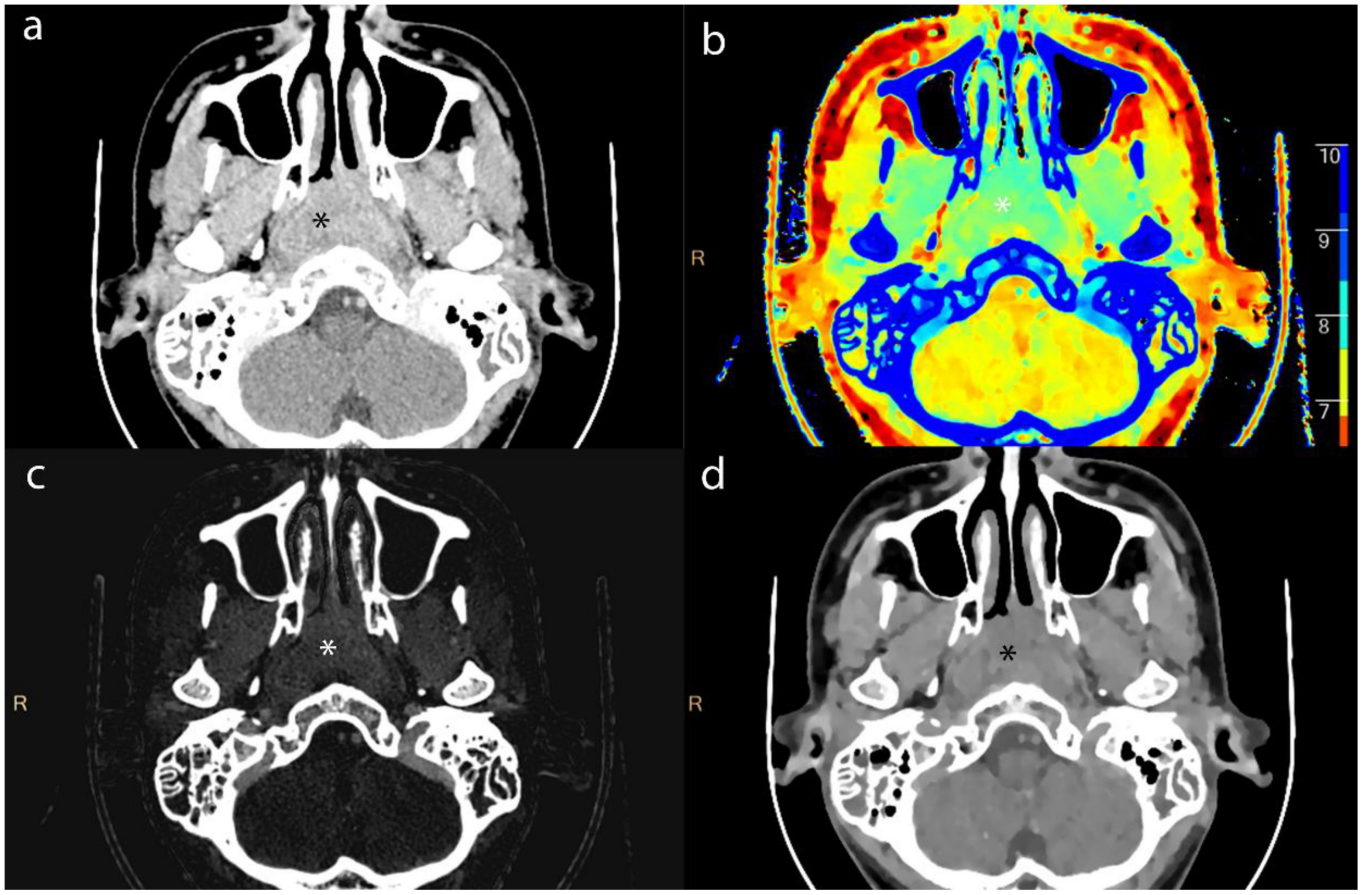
Axial contrast-enhanced CT images of lesion (*) from a 16-year-old male patient with NPC. (a) shows the short-axis diameter and CT value of the lesion. (b)- (d) shows the Zeff maps, Iodine no water maps and ED maps of lesion.
